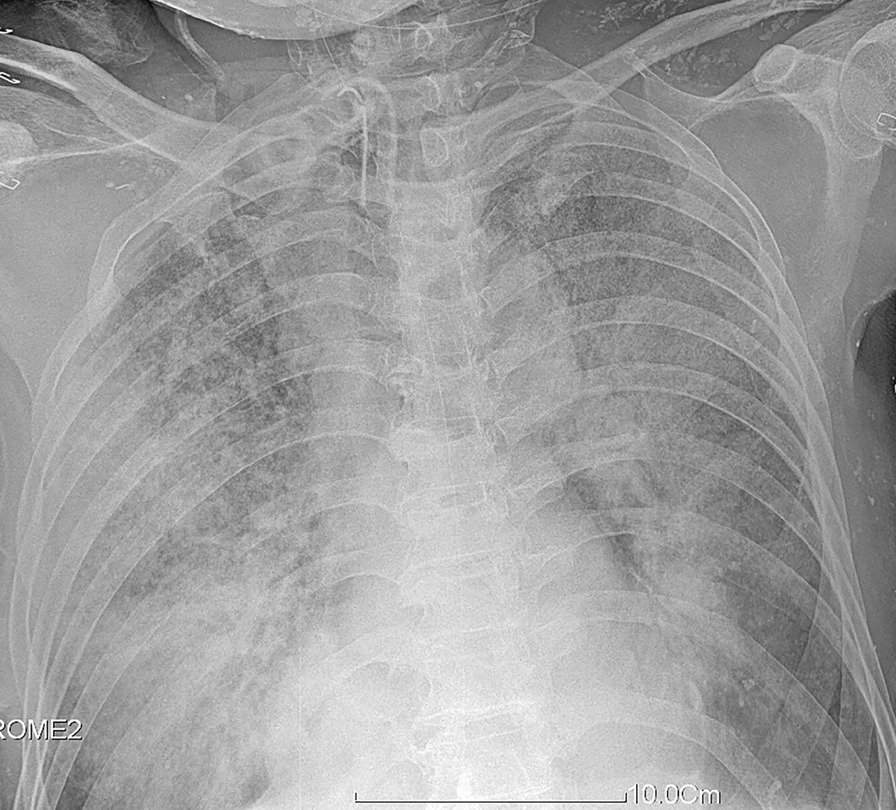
Chest X-ray showing large patellar high-density shadows in both lungs, significantly reduced pulmonary transmittance, and a widened hilum of both lungs
